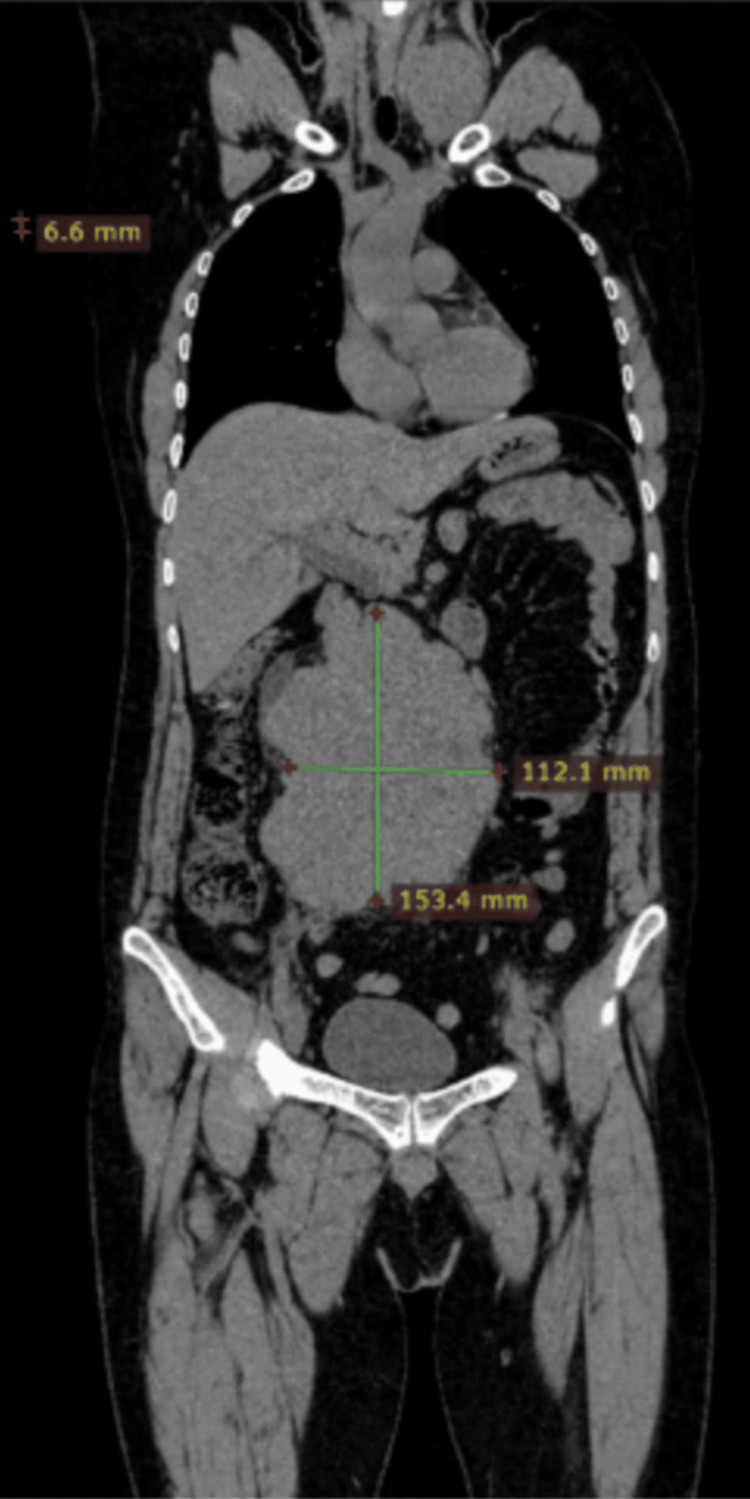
Coronal image of a CT scan showing a mass of 11 cm in the abdomen CT: computed tomography
